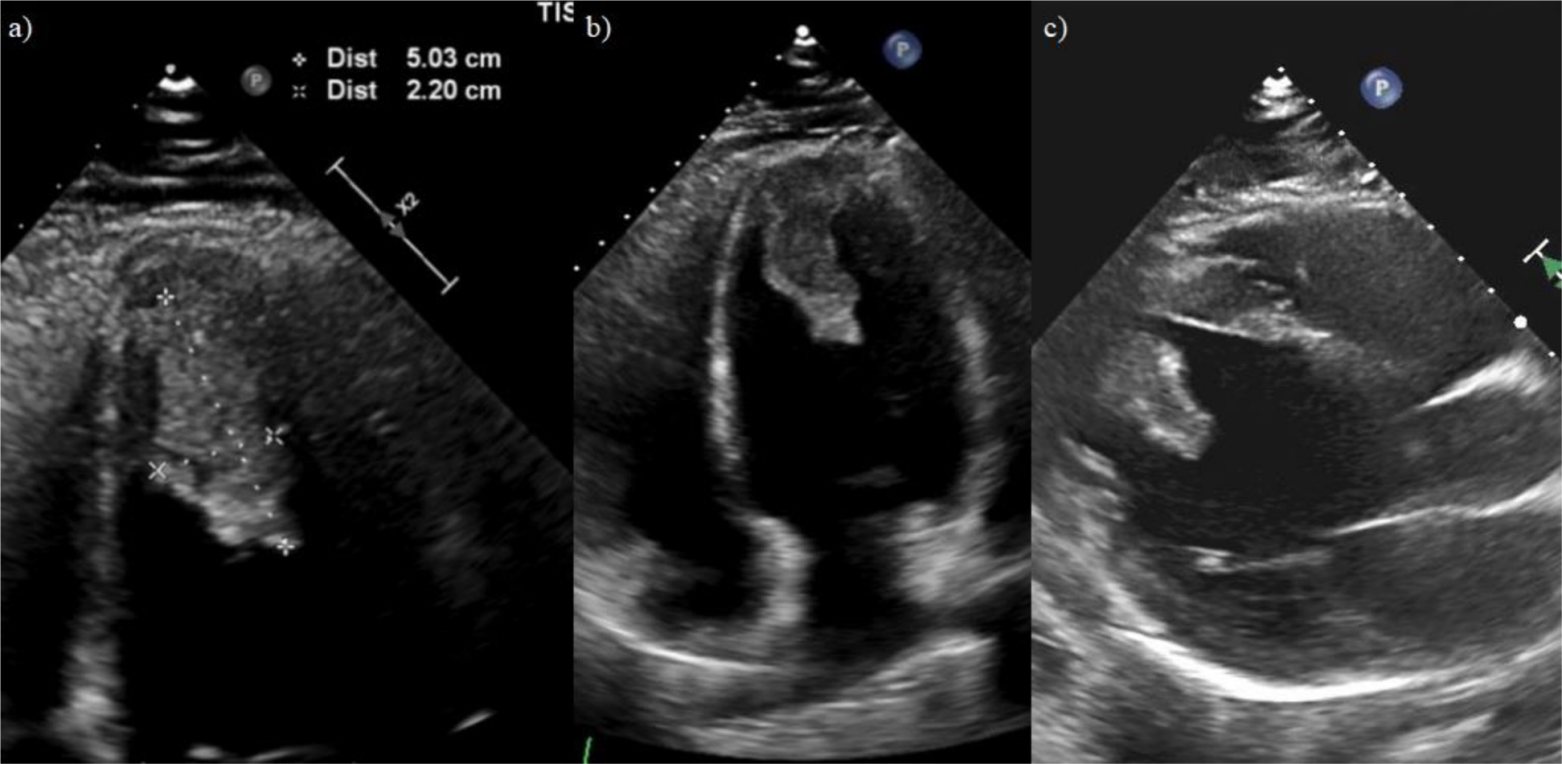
Transthoracic echocardiogram without contrast. (A) Zoomed apical four-chamber view demonstrating the LV apical mass with dimensions of 5 × 2.2 cm. (B) Apical four-chamber view demonstrating a hyperechoic, mobile, and pedunculated LV apical mass with margins distinct from the endocardial edge and defined contours consistent with thrombus. (C) Left parasternal long-axis view demonstrating the LV apical mass extending into the midcavity.
